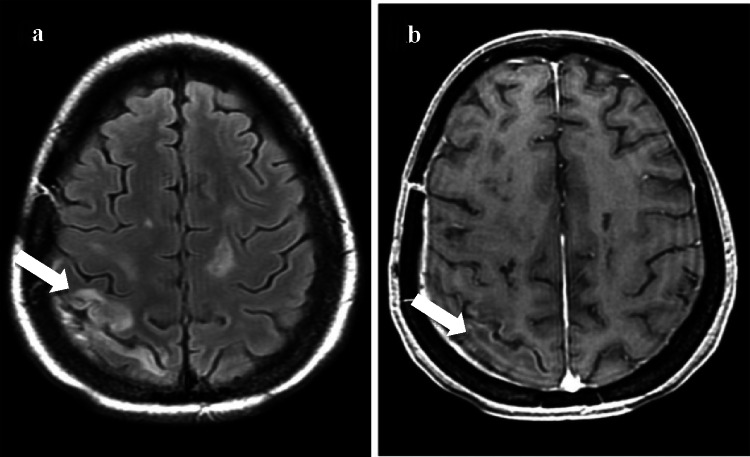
a Post-contrast T2/FLAIR imaging shows new onset altered signal intensity along the post-central gyrus 14 weeks after starting chemotherapy. b Post-contrast T1W image shows subtle leptomeningeal enhancement along post-central sulcus and superior parietal lobe
